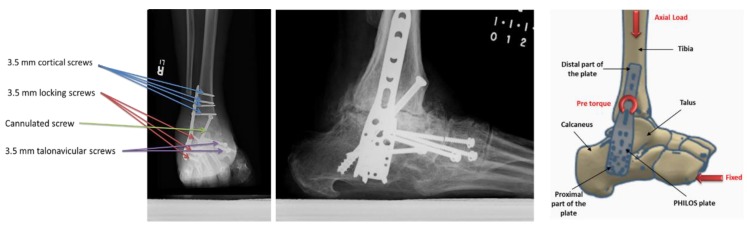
X-ray images of the PHILOS construct after two years showing the different types of screws used in fixation (cannulated and talonavicular screws were excluded from this study) (left and middle). The 3D model (right).
